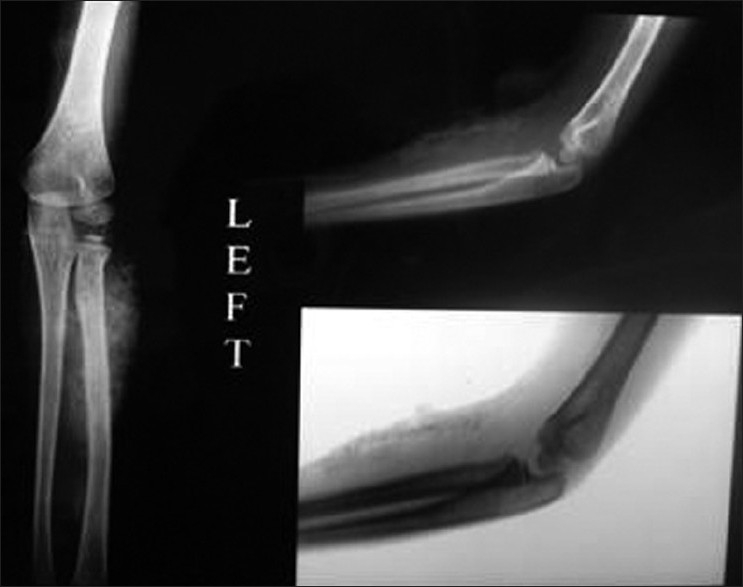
X-ray of left forearm with elbow joint (anteroposterior and lateral views) showing ossification in the dermis and subcutaneous tissue in February 2010
